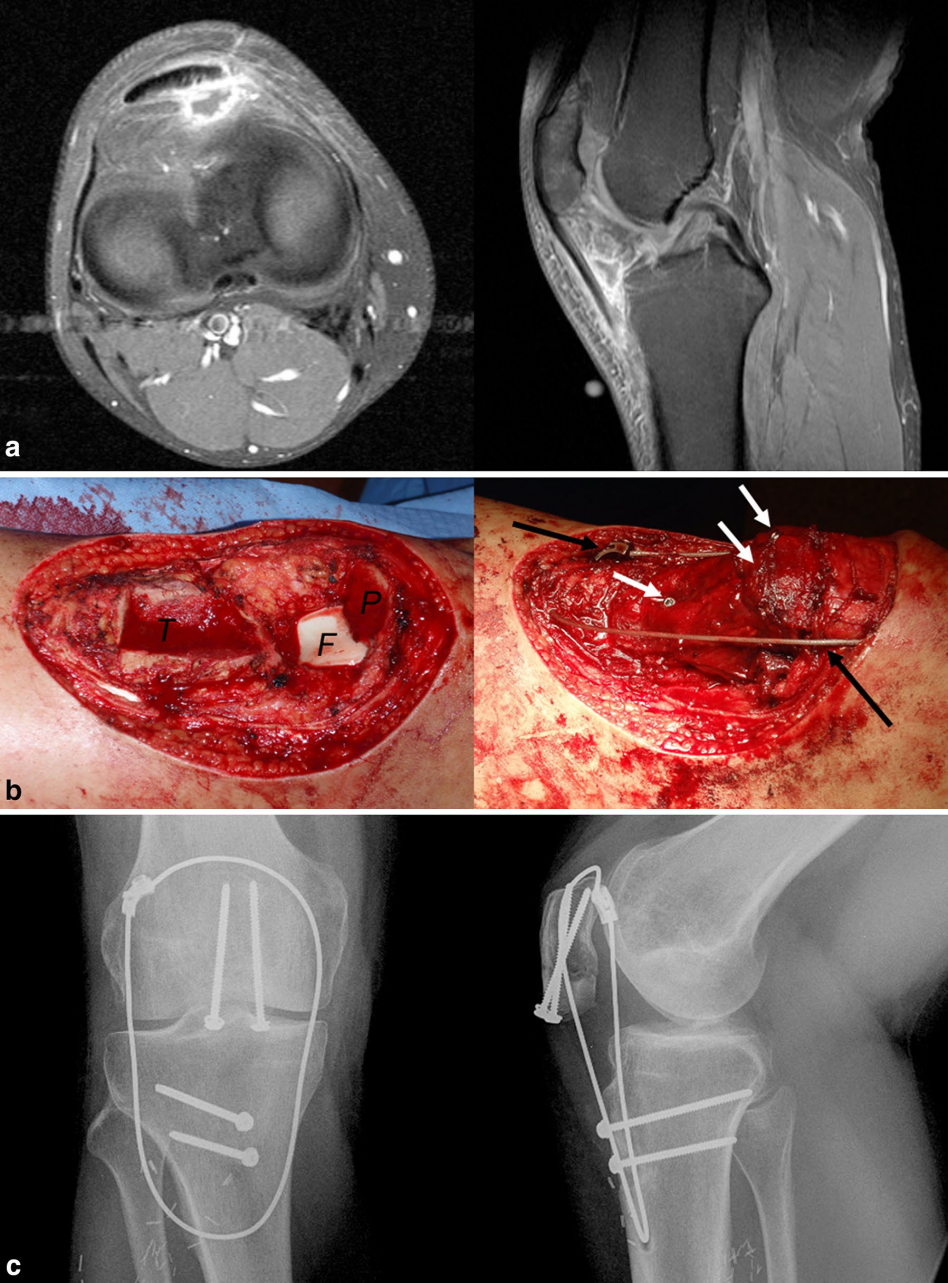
Radiologic studies and photographs of patient 1. a T1 gadolinium-enhanced magnetic resonance images at the first presentation show inflammation in the infrapatellar fat pad after unplanned excision. b
